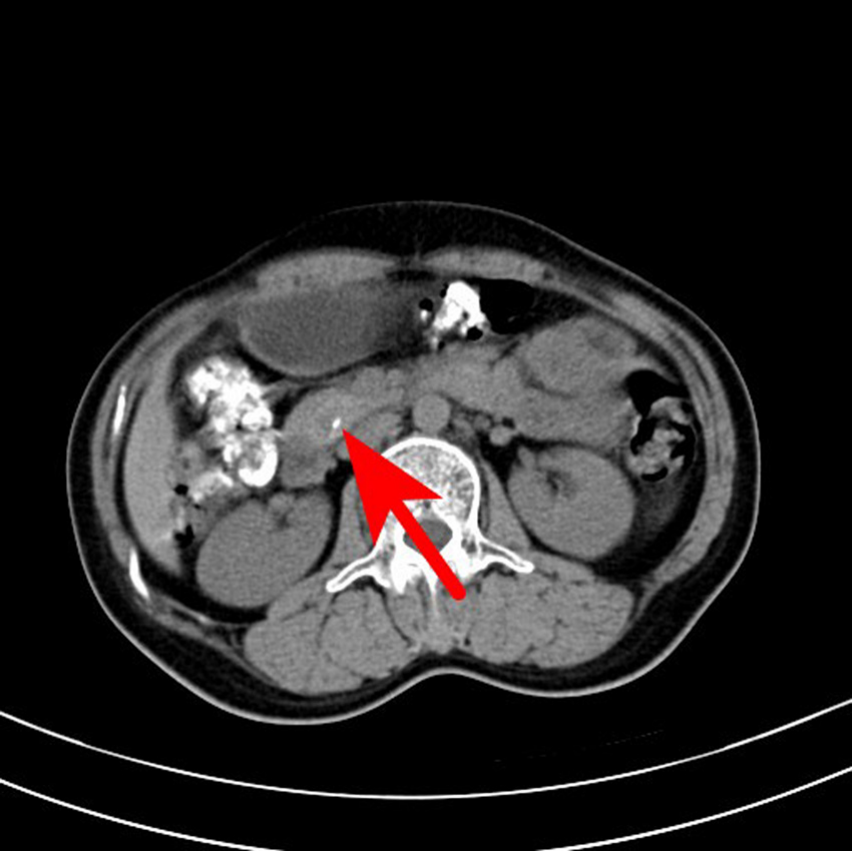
CT scan of the abdomen of the patient. The arrow points to a nodular slightly high-density shadow on the head of the pancreas, with a maximum transverse diameter of about 19 mm and a clear boundary, and a small saccular low-density shadow and a punctate calcification shadow.
